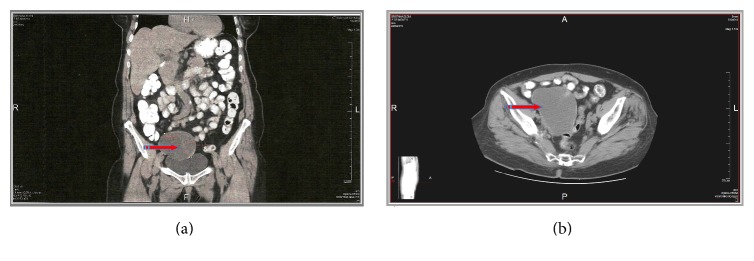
Abdominal CT revealed a cyst sized 100 × 80 mm with signs of calcification in the area of the right ovary (arrows).
